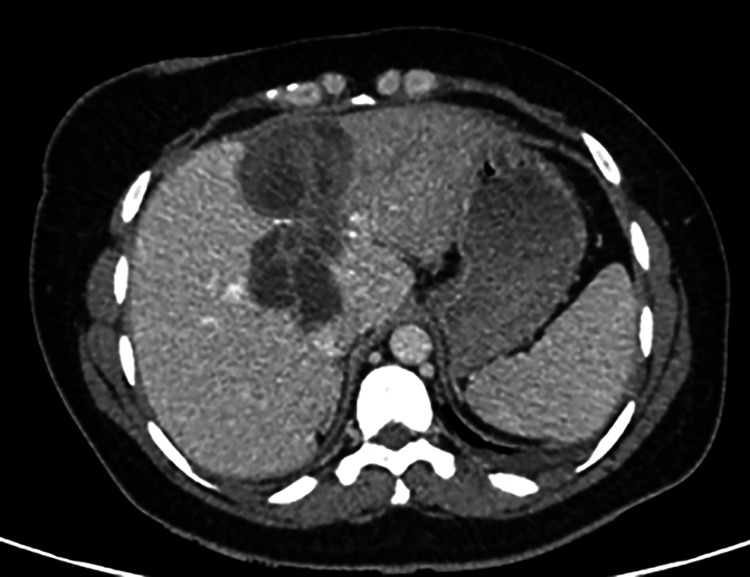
CT scan detected two hydatid cysts of the liver.
